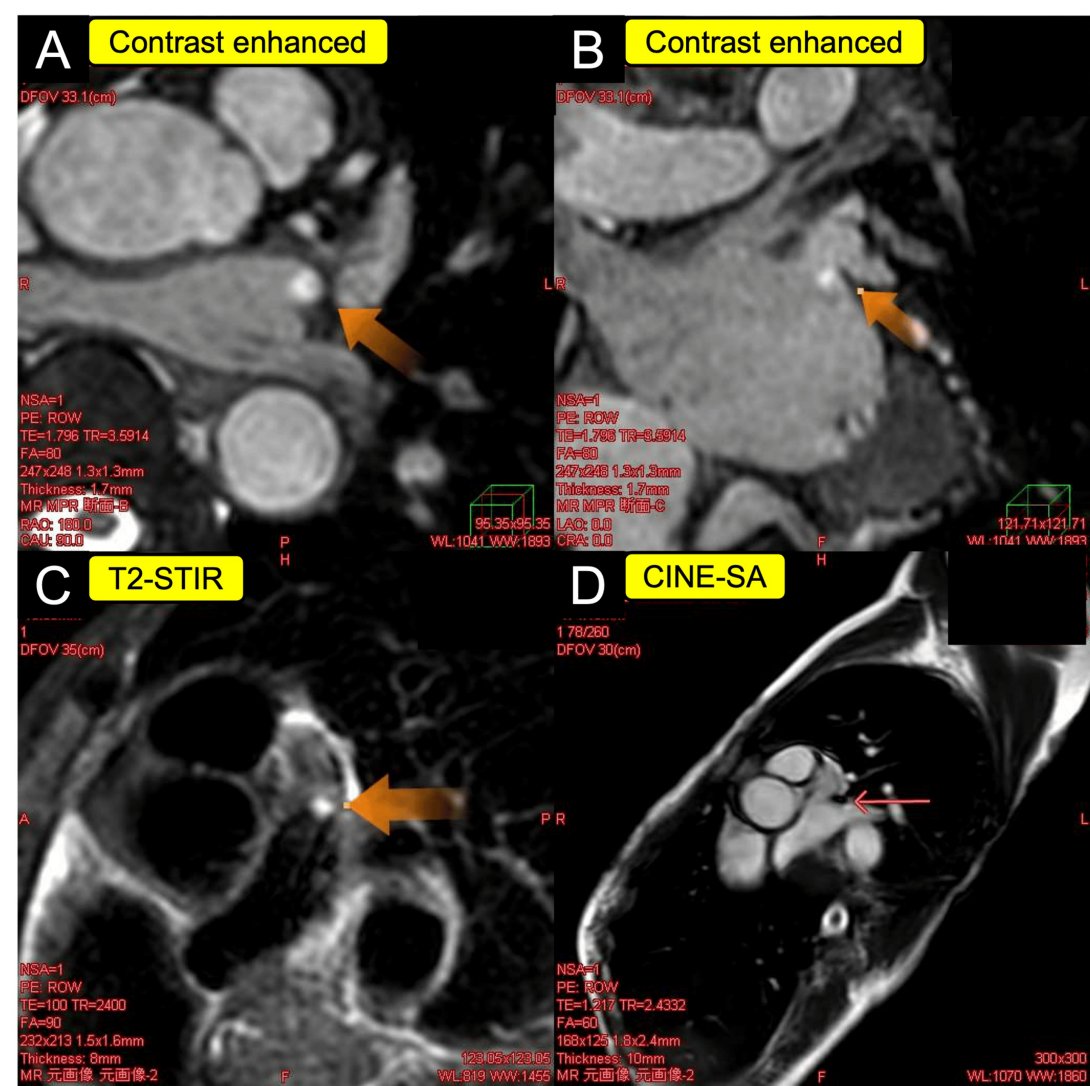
Cardiac magnetic resonance imaging of the case (A, B) Contrast enhanced showed the magnetic resonance imaging enhancement of the mass (orange arrow). (C) T2-STIR showed the hyperintense mass (orange arrow). (D) Cine SA showed the isointense mass (arrow). T2-STIR: T2-weighted short tau inversion recovery; SA: short-axis
